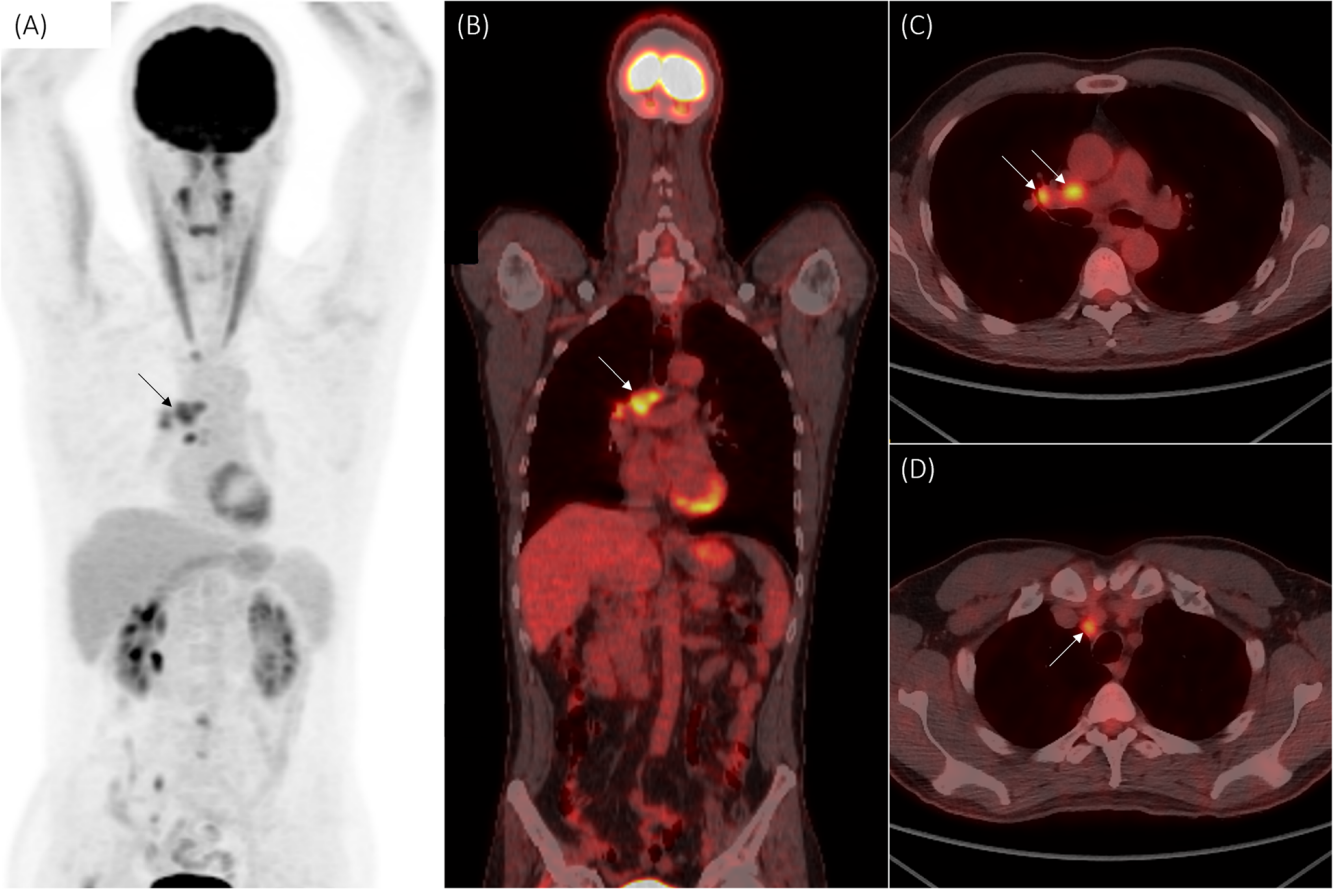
FDG-PET body scan: multiple lymph nodes with FDG-avid uptake in right subcarinal and right hilar (A-C) and superior right paratracheal (D) areas. FDG = fluorodeoxyglucose, PET = positron emission tomography
